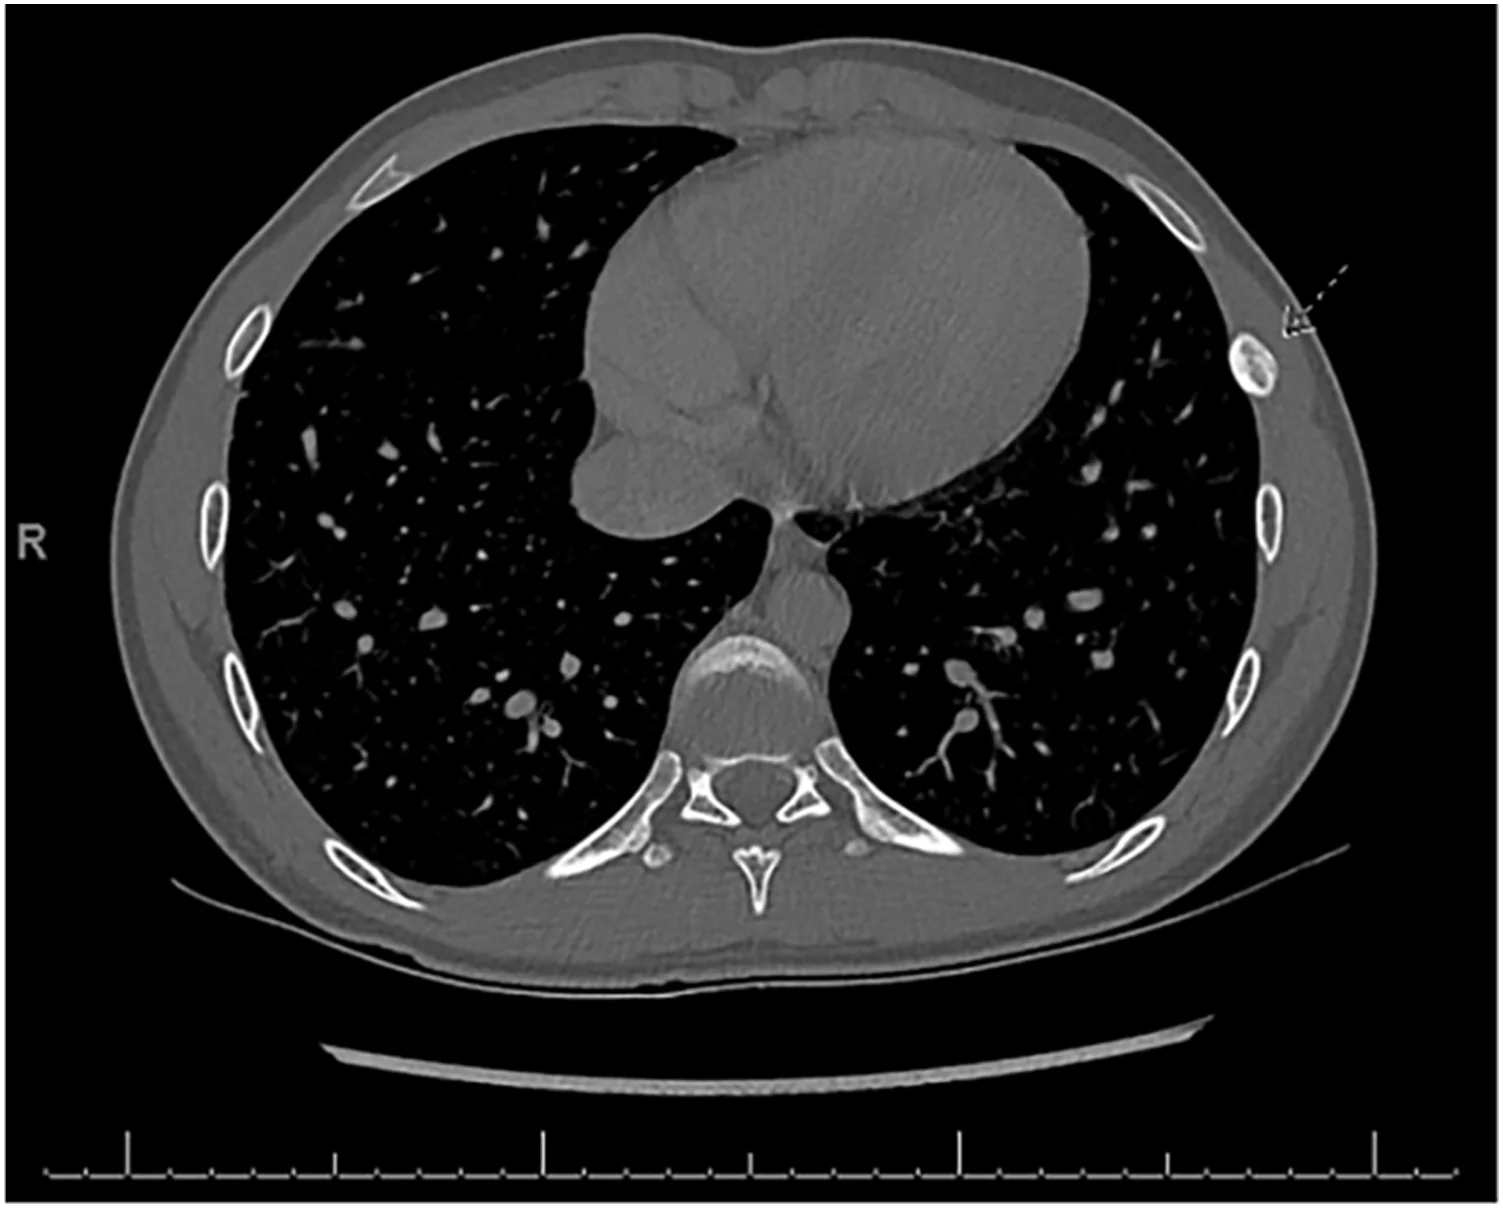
Computed tomography of the chest of T5 and T6 fractures. The arrow shows the fracture. (R, right [part of the CT scan].)
